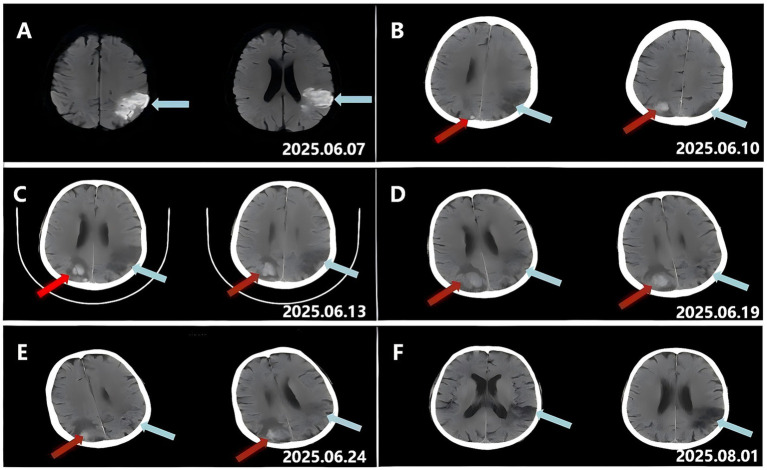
The magnetic resonance imaging or computed tomography results of brain. (A) Cranial magnetic resonance imaging at the initial occurrence of neurological complications. A large left-sided intracerebral infarction. (B) Computed tomography (CT) scanning with the brain on 5th day. A minor right-sided intracerebral bleeding and a stable left-sided intracerebral infarction. (C) CT scanning with the brain on 7th day. An increased right-sided intracerebral bleeding and a stable left-sided intracerebral infarction. (D–F) CT scanning with the brain on 15th, 20th, and 58th day. An improved right-sided intracerebral bleeding and a stable left-sided intracerebral infarction. Blue arrows indicate the location of the cerebral infarction. Red arrows indicate the location of the cerebral hemorrhage.
